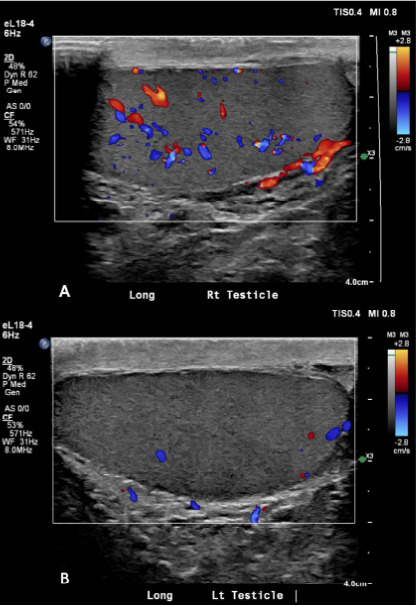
Testicular ultrasound with Doppler: A) right testicle showing normal flow; B) left testicle showing decreased blood flow concerning for torsion.
